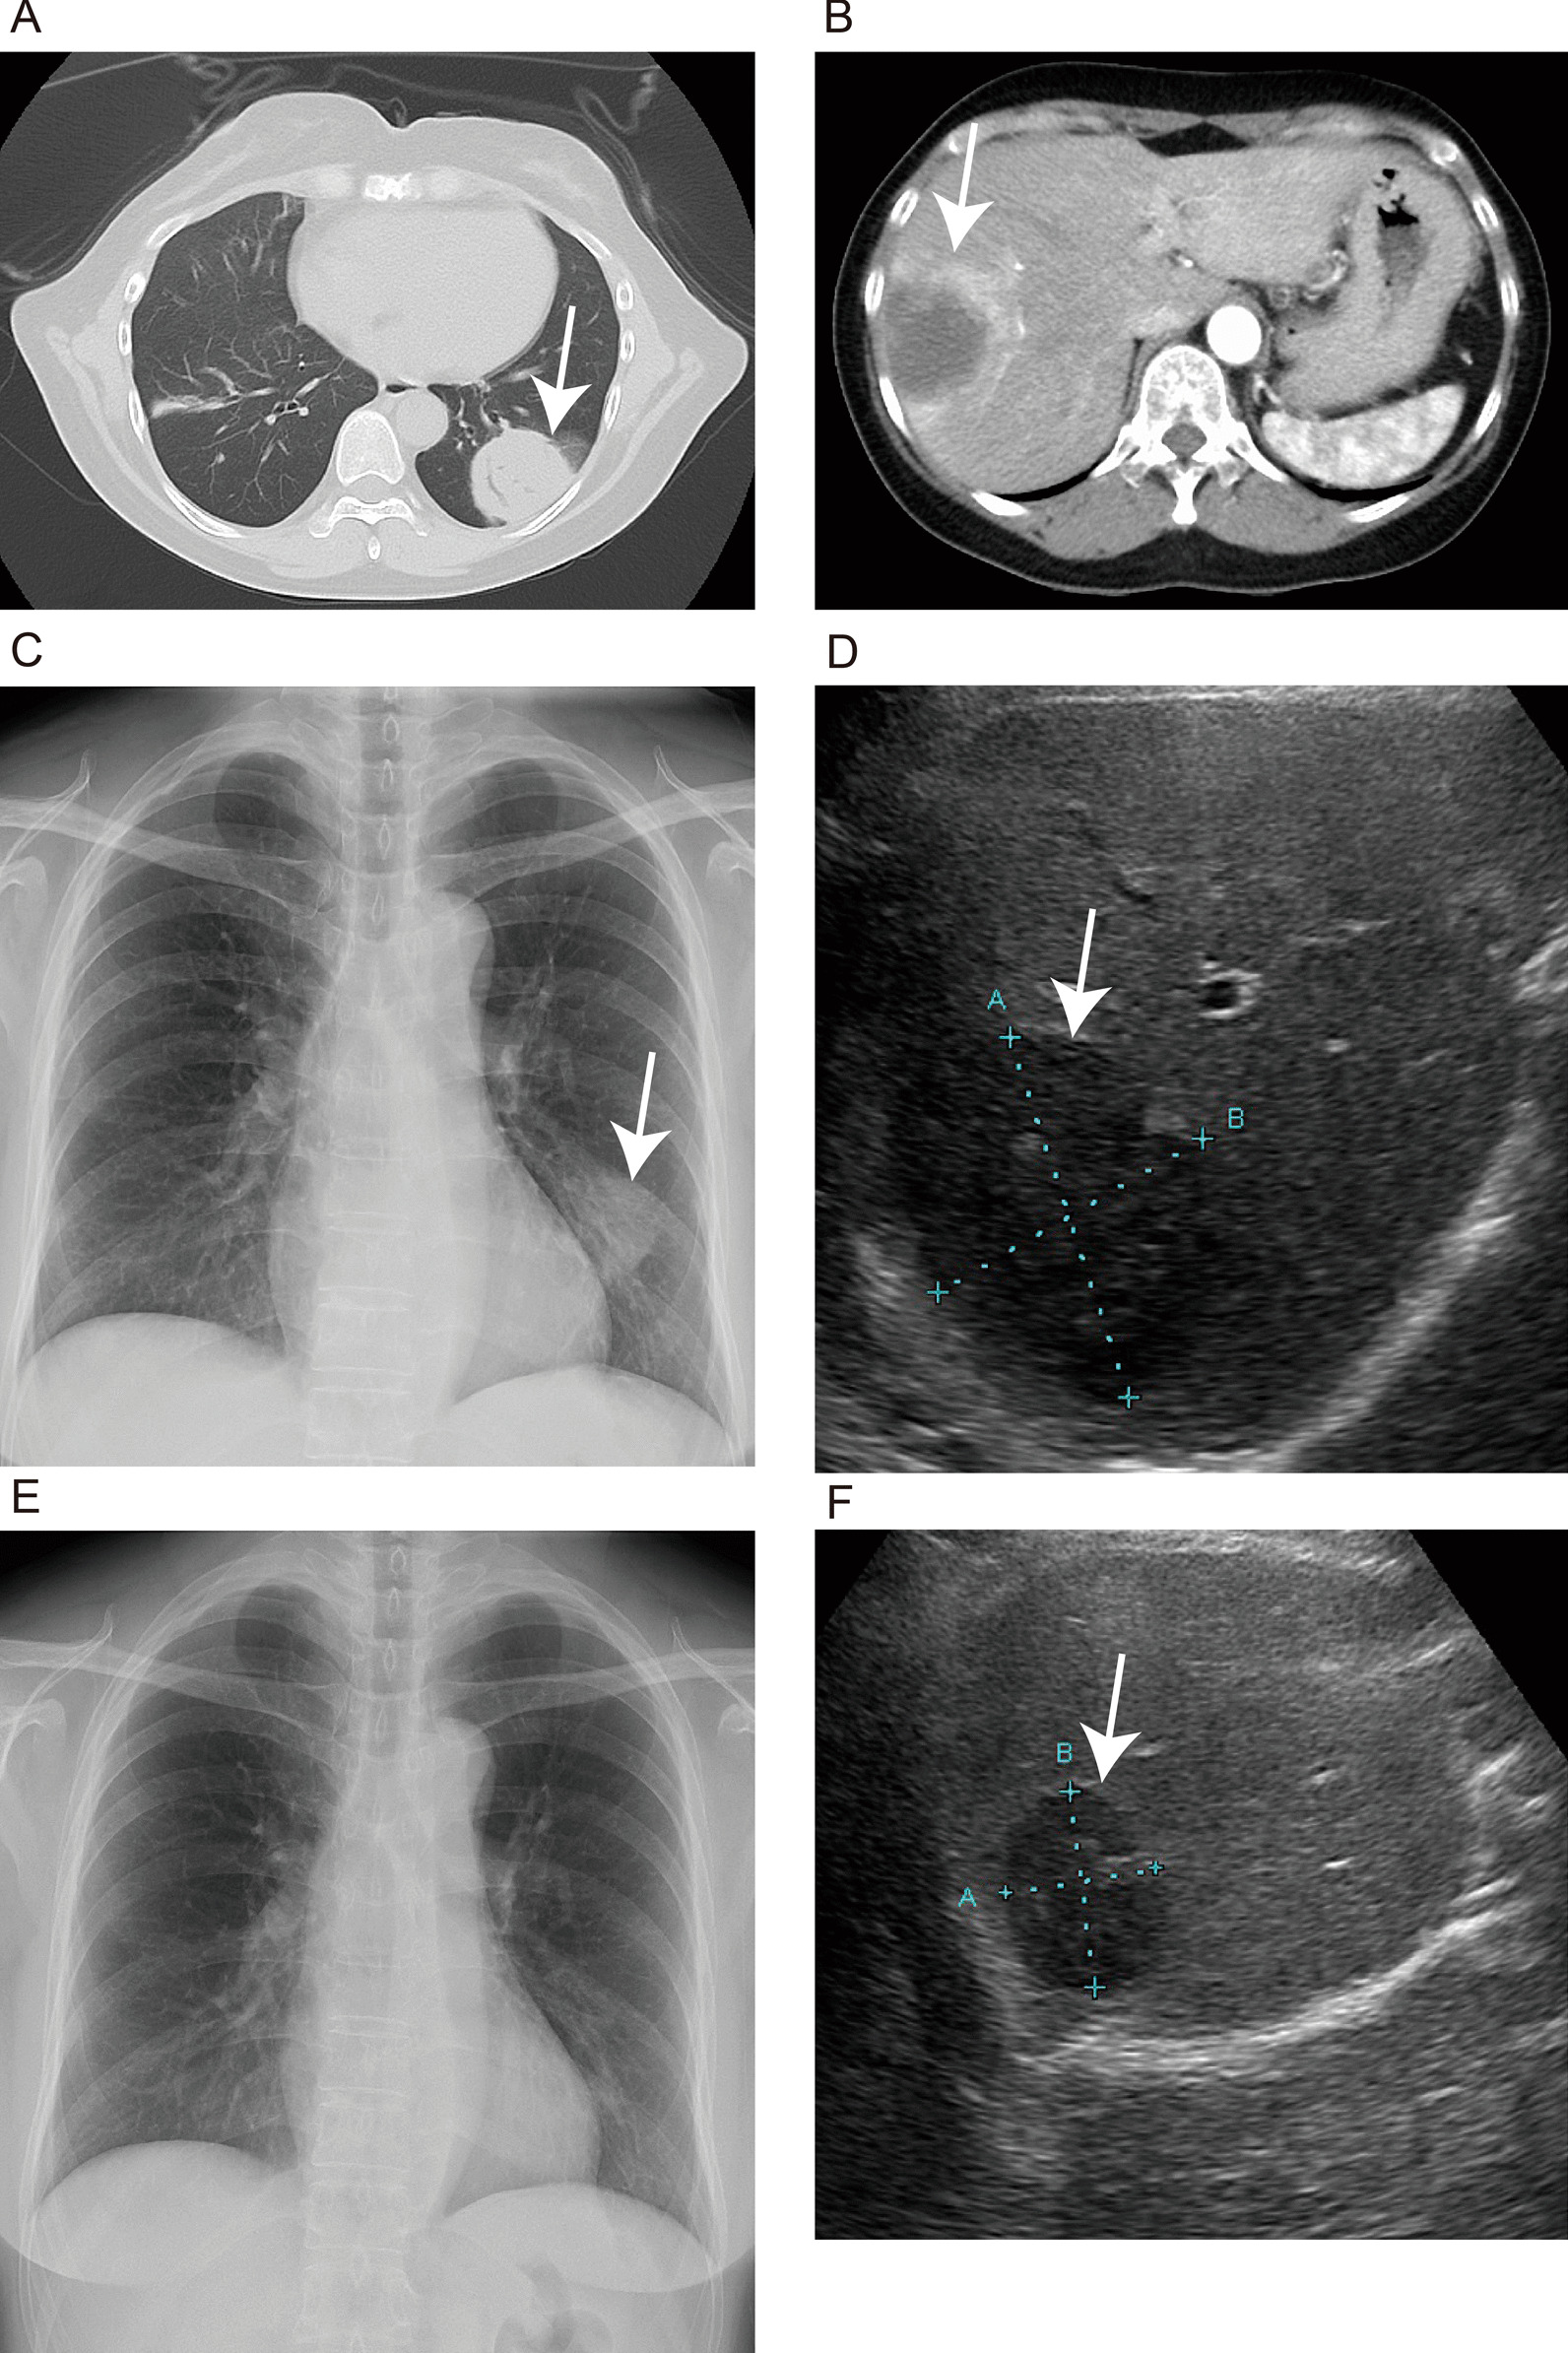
Appearance of lung and hepatic masses by computed tomography (CT), chest X-ray, and ultrasonography (US). A large lung nodule is shown on CT at the initial visit (A, arrow) and chest radiography at 4 weeks (C, arrow) and 3 months (E). A large hepatic nodule is shown on enhanced CT at the initial visit (B, arrow) and US at 4 weeks (D, arrow) and 3 months (F, arrow)
